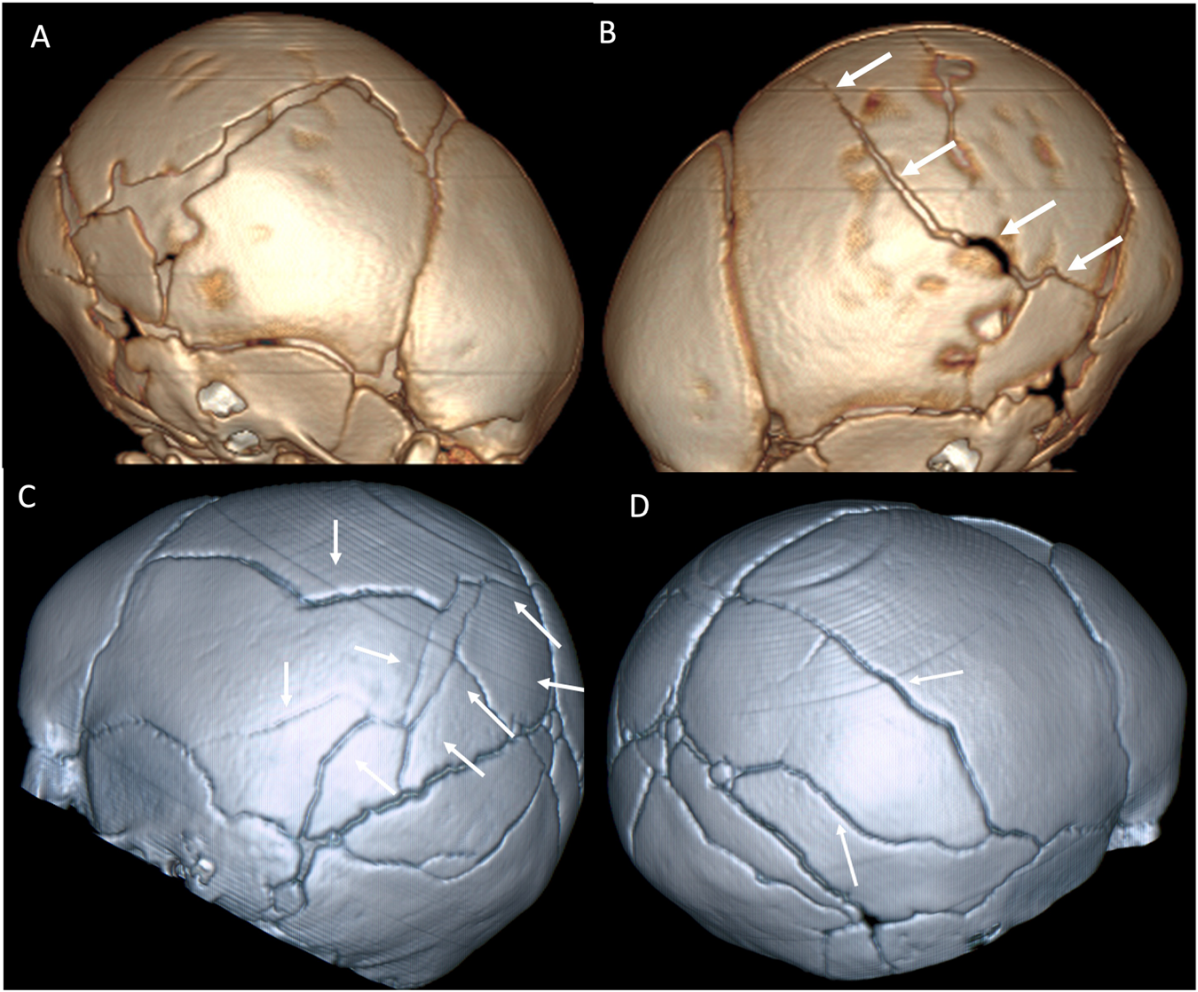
Skull fractures on 3-D reconstructed CT images. A, B Complex fractures (arrows) of the right parietal bone (A) with a displaced quadrangular-shaped fragment (asterisk in A) and linear fracture (arrows) of the left parietal bone (B) in a 6-week-old boy. C, D Complex fractures of the left (C) and right (D) parietal bones (arrows) in a 5-month-old boy
